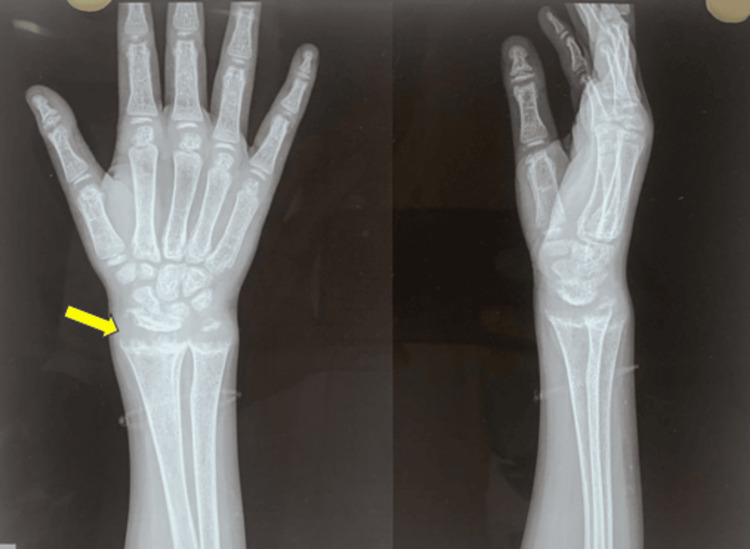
X-ray of right hand X-ray of right hand showing features of rickets in the form of splaying, fraying, and cupping (arrow).
